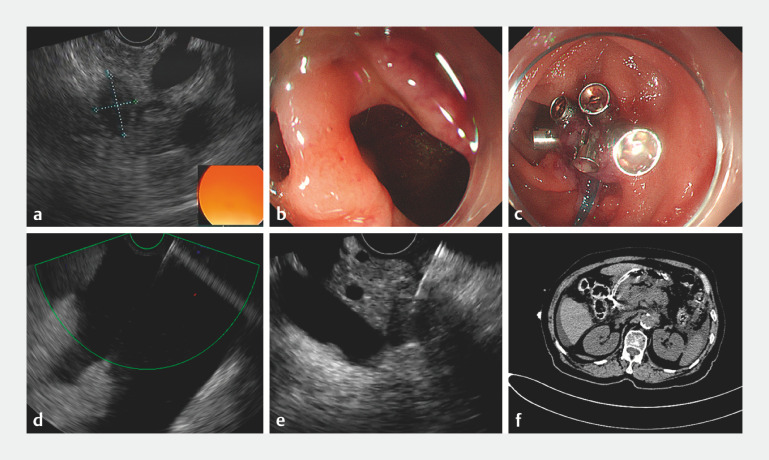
Management of endoscopic ultrasound (EUS)-related duodenal perforation during pancreatic cancer sampling.
